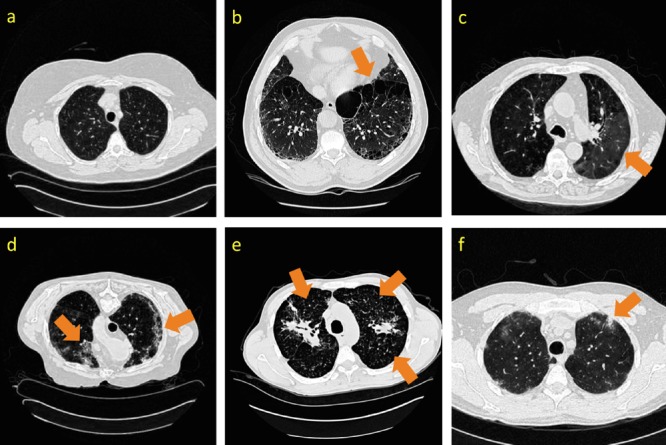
Some examples of CT image slices with six lung tissue types in the ILD dataset [37]. Disease tissue types are located with dark orange arrows. (a): healthy; (b): emphysema; (c): ground glass; (d): fibrosis; (e): micronodules; (f): consolidation.
